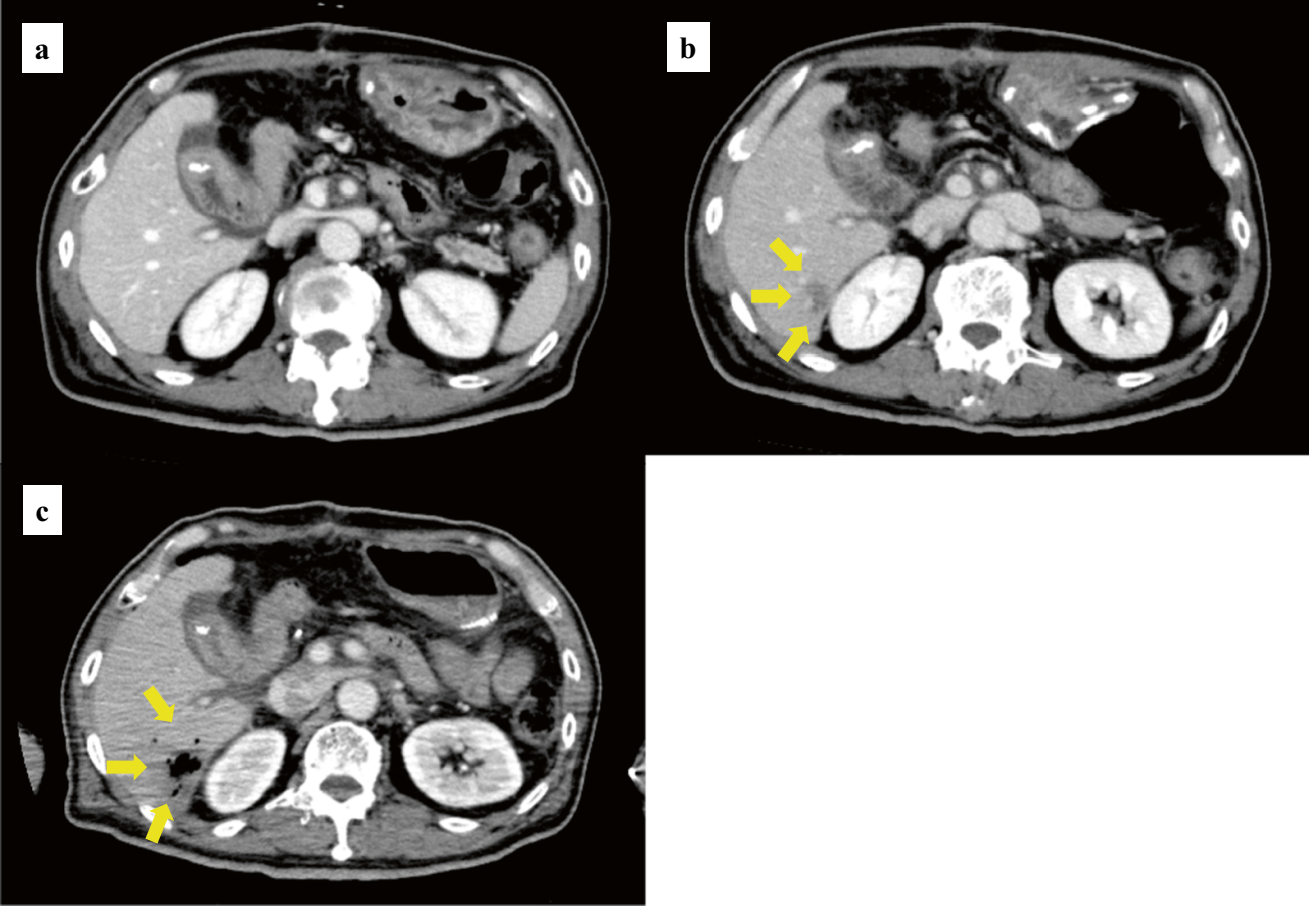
Computed tomography findings over time. a Postcontrast image showing no abnormal findings on POD 14, 2 days before discharge. b Image obtained when the patient was readmitted on POD18 showing an irregular, 20 mm, low-density area in the S6 region of the liver. c Image obtained 8 h after admission showing that the affected area has transformed into a gas-producing abscess
